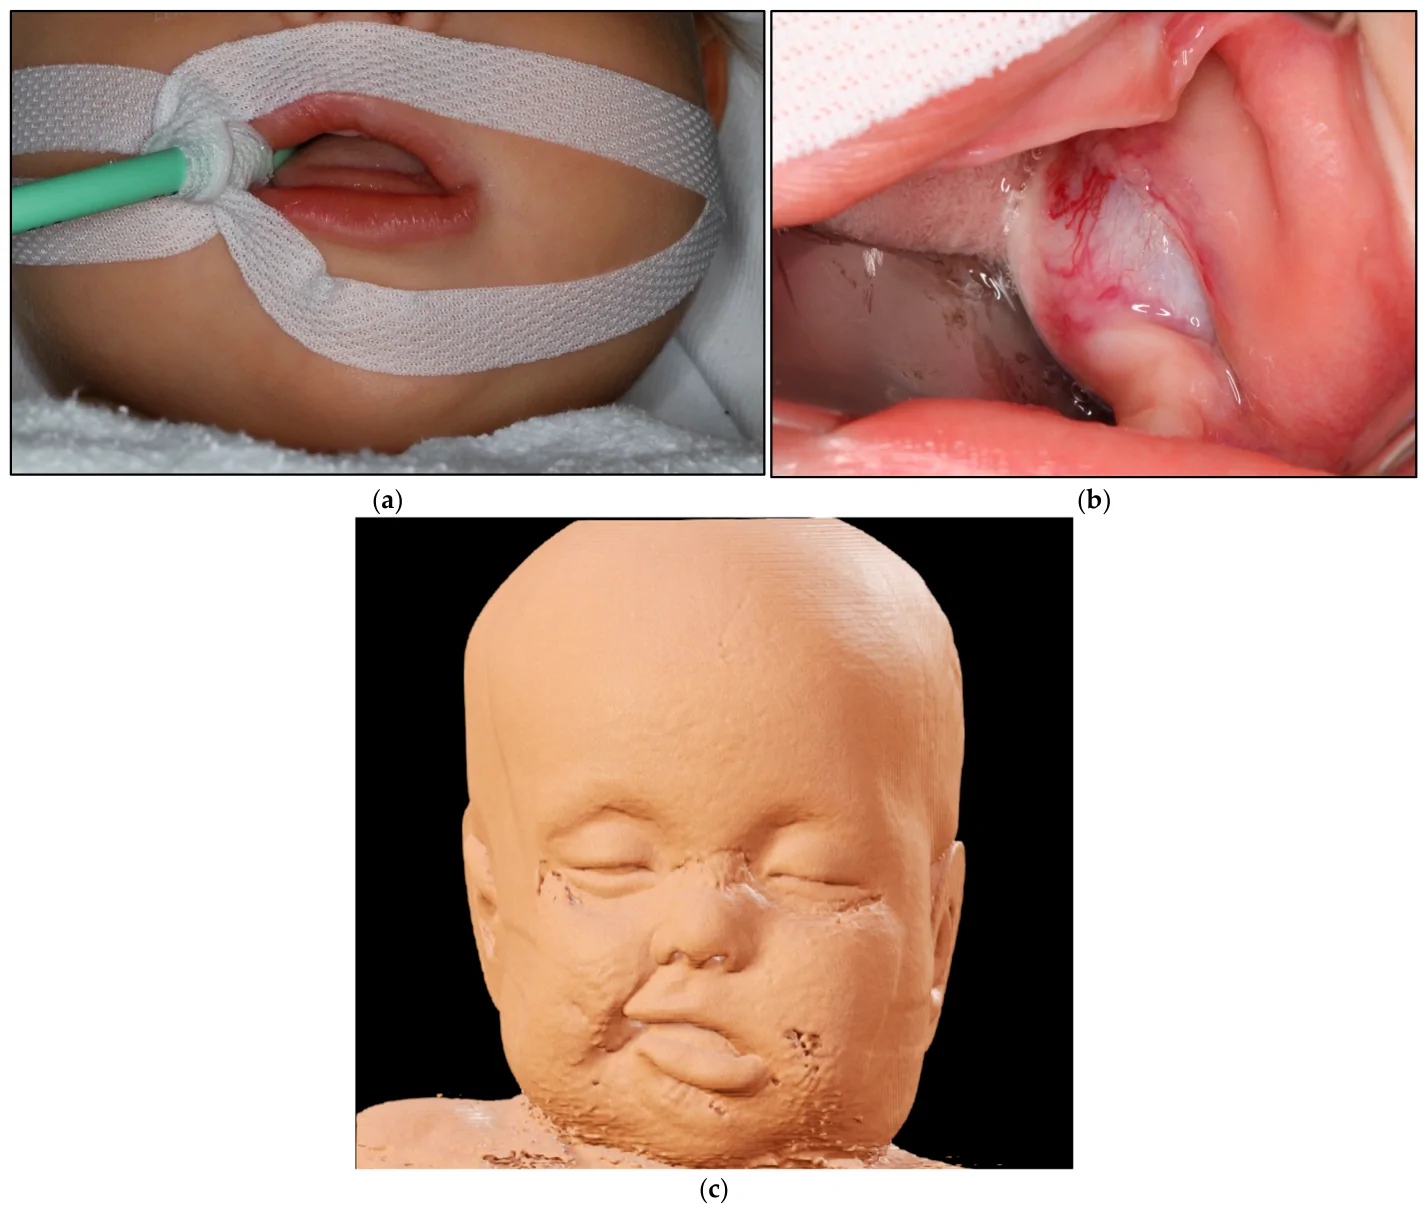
(a,b). Buccal swelling and mandibular mass on the left side. (a) Presentation with suspected parotitis; painless swelling of the cheek soft tissues. (b) Firm swelling of the edentulous mandible with a pearlescent surface, neovascularization, and indistinct borders. (c) Three-dimensional MRI reconstruction demonstrating the left-sided facial asymmetry associated with the mandibular lesion. Reconstruction generated using Brainlab^®^ Software Elements 7.0 (Brainlab AG, Munich, Germany).
